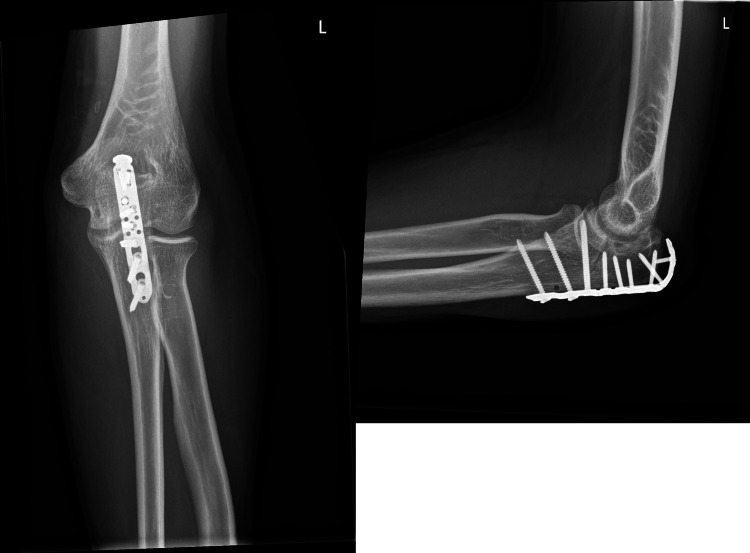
Six-week postoperative X-ray of the olecranon A six-week postoperative X-ray in the coronal (a) and sagittal (b) views of the fixed olecranon demonstrating progressive bone healing and proper alignment of the elbow.
